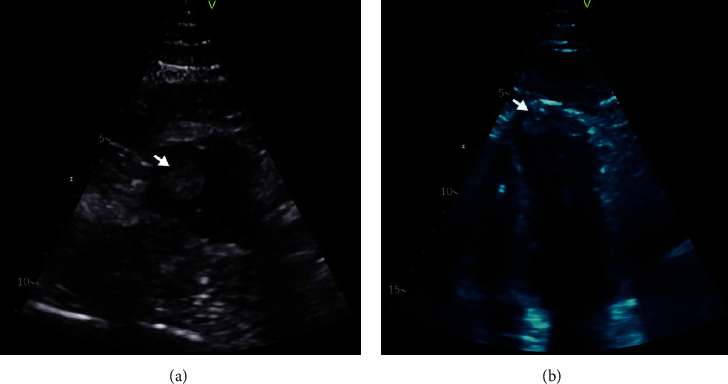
Post device upgrade ECHO. (a) Parasternal short axis view and (b) apical 4-chamber view of transthoracic echocardiogram demonstrating the left ventricular apical thrombus (arrow).
